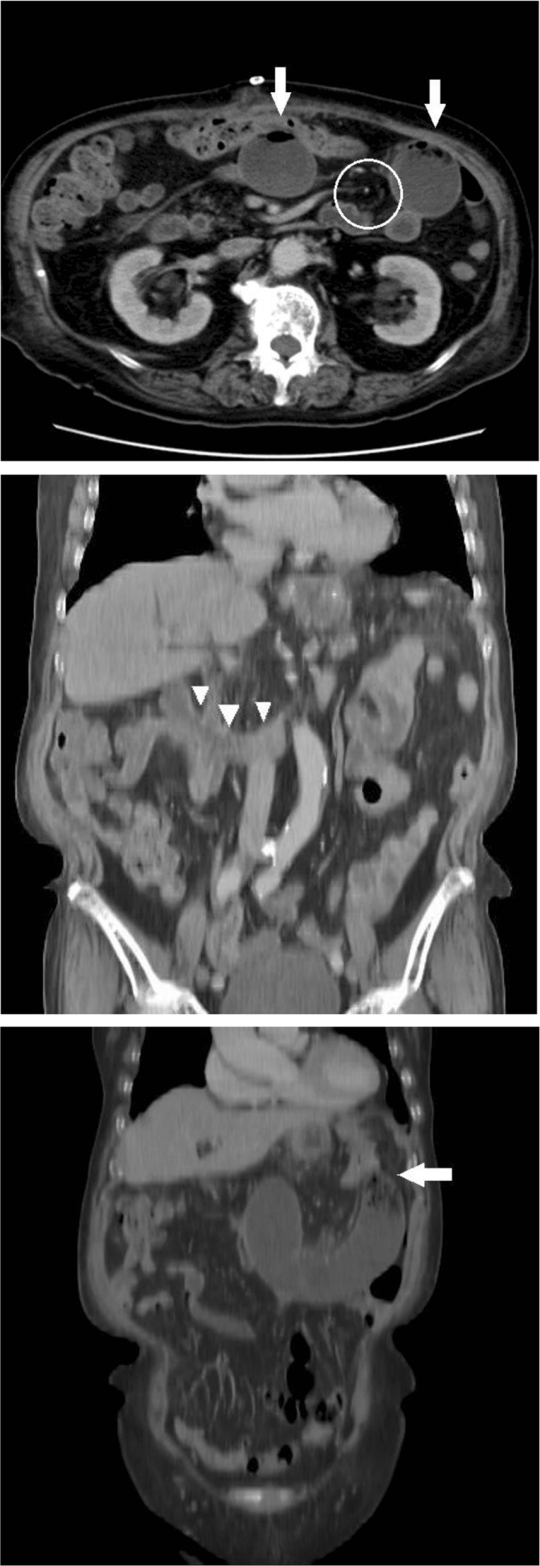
CT scan findings. Axial CT scan shows crowding of the main mesenteric trunk to the left and distention of the elevated jejunum (*white arrow*). A swirled appearance of the mesenteric fat and vessels was found (*white circle*). Coronal CT scan shows no distension of the jejunum around the Treitz ligament (*white arrowheads*). The obstruction was located at the elevated jejunum about 10 cm from the Y anastomosis. *White arrow* shows the kinking point of elevated jejunum in coronal CT scan
